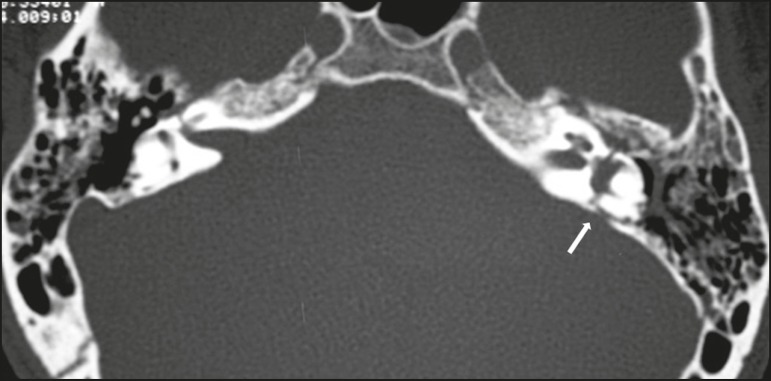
Fracture. Axial CT, with a bone window, showing a transverse
